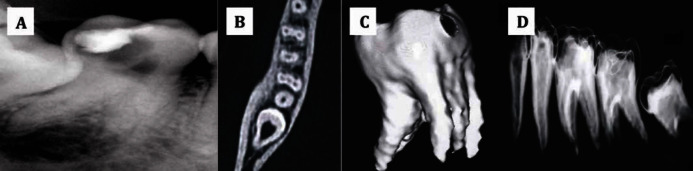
A) Pre-operative diagnostic radiograph; B) spiral CT scan showing the presence of three independent mesial canals; C) three dimensional reconstruction of the mandibular second molar with 3 mesial roots; D) spatial orientation of the mandibular second molar.
